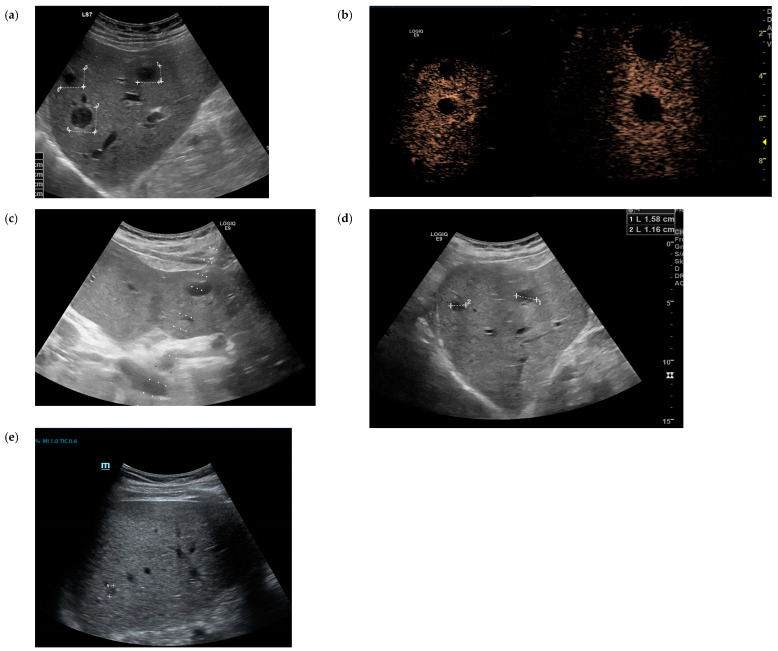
Abdominal and contrast-enhanced ultrasound (CEUS) of the liver. On admission: the liver with multiple small echo-poor lesions sharply delineated from the surrounding tissue (a); CEUS: no enhancement of the lesions, but hyperenhancement in the periphery of the abscess (b). Ultrasound-guided diagnostic punction of one lesion to gain 3 ml pus (c). Progress control after 2 weeks (d) and 6 weeks (e). The diameter of the multiple echo-poor lesions decreased over 6 weeks under therapy.
